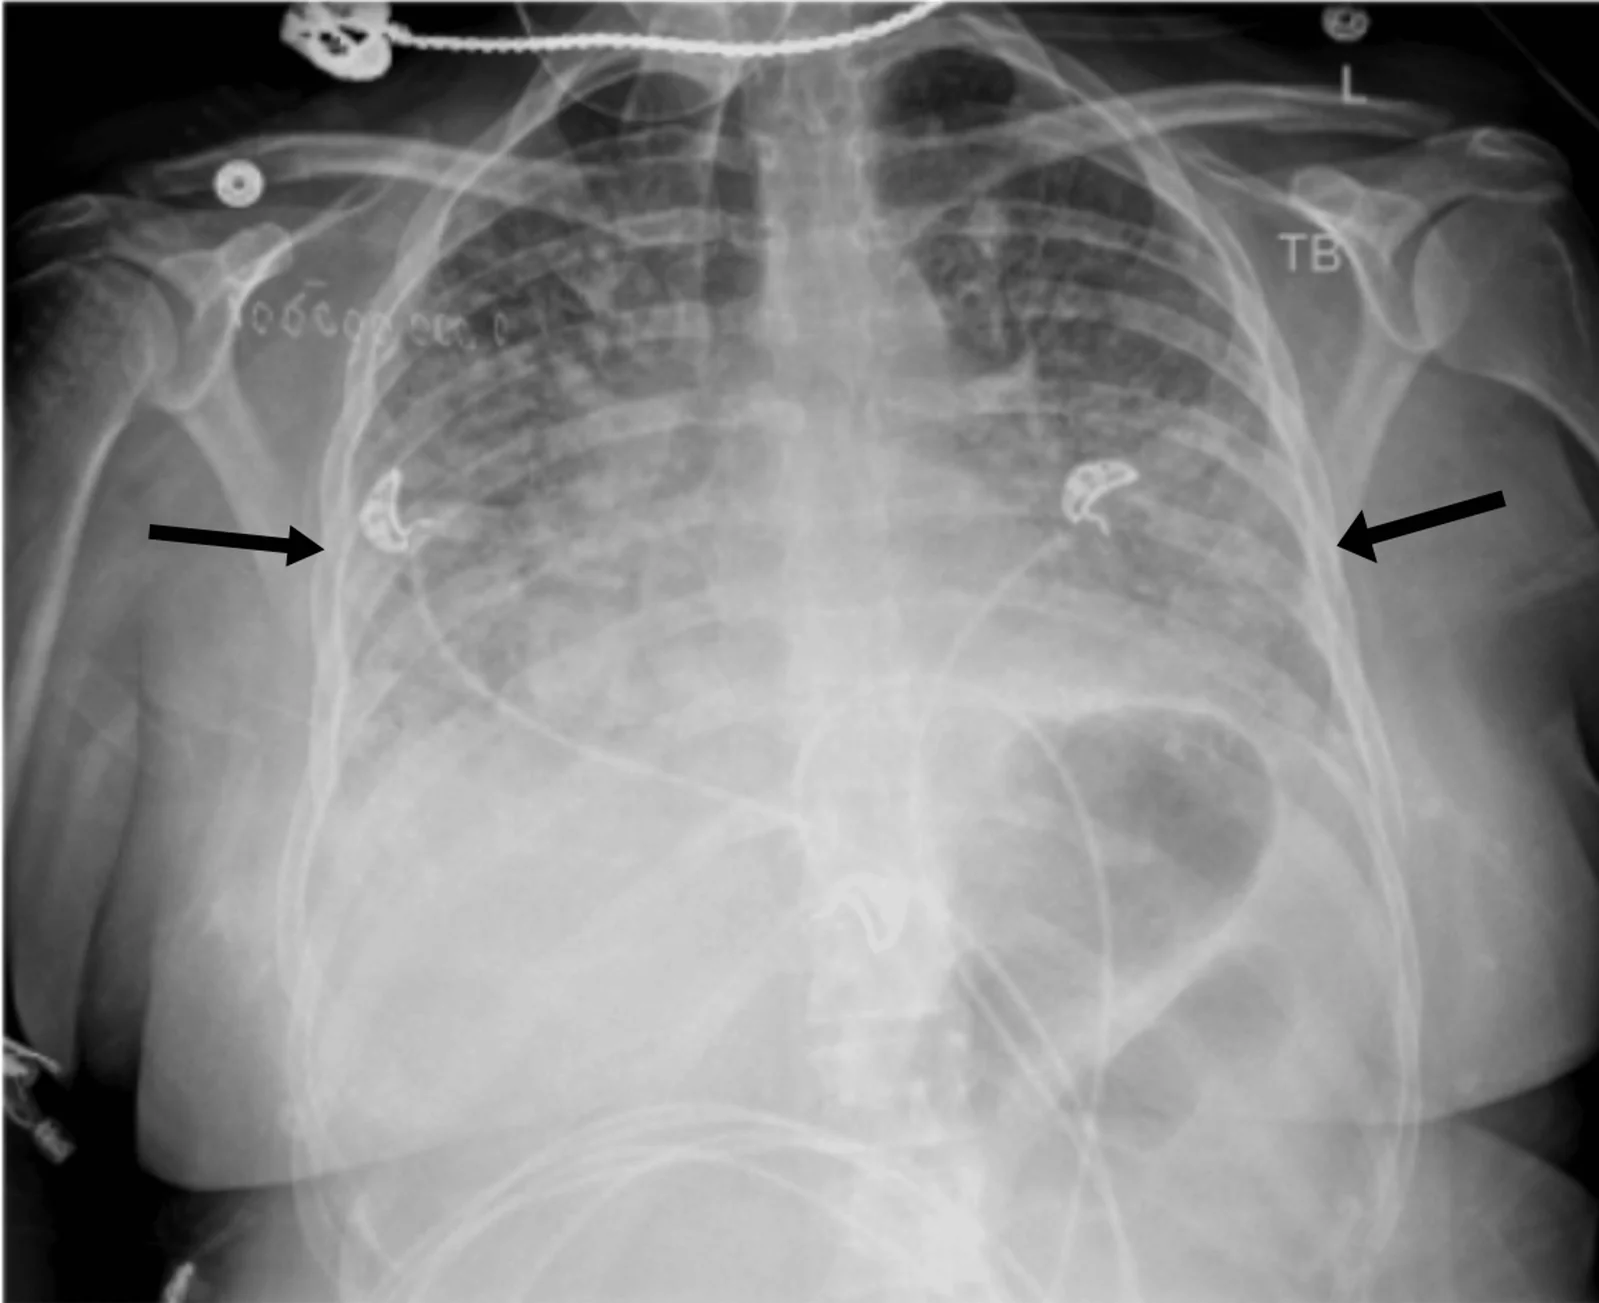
Chest X-ray demonstrating persistent, diffuse bilateral opacities (black arrow) at readmission, almost one month following patient's initial thrombectomy surgery.
